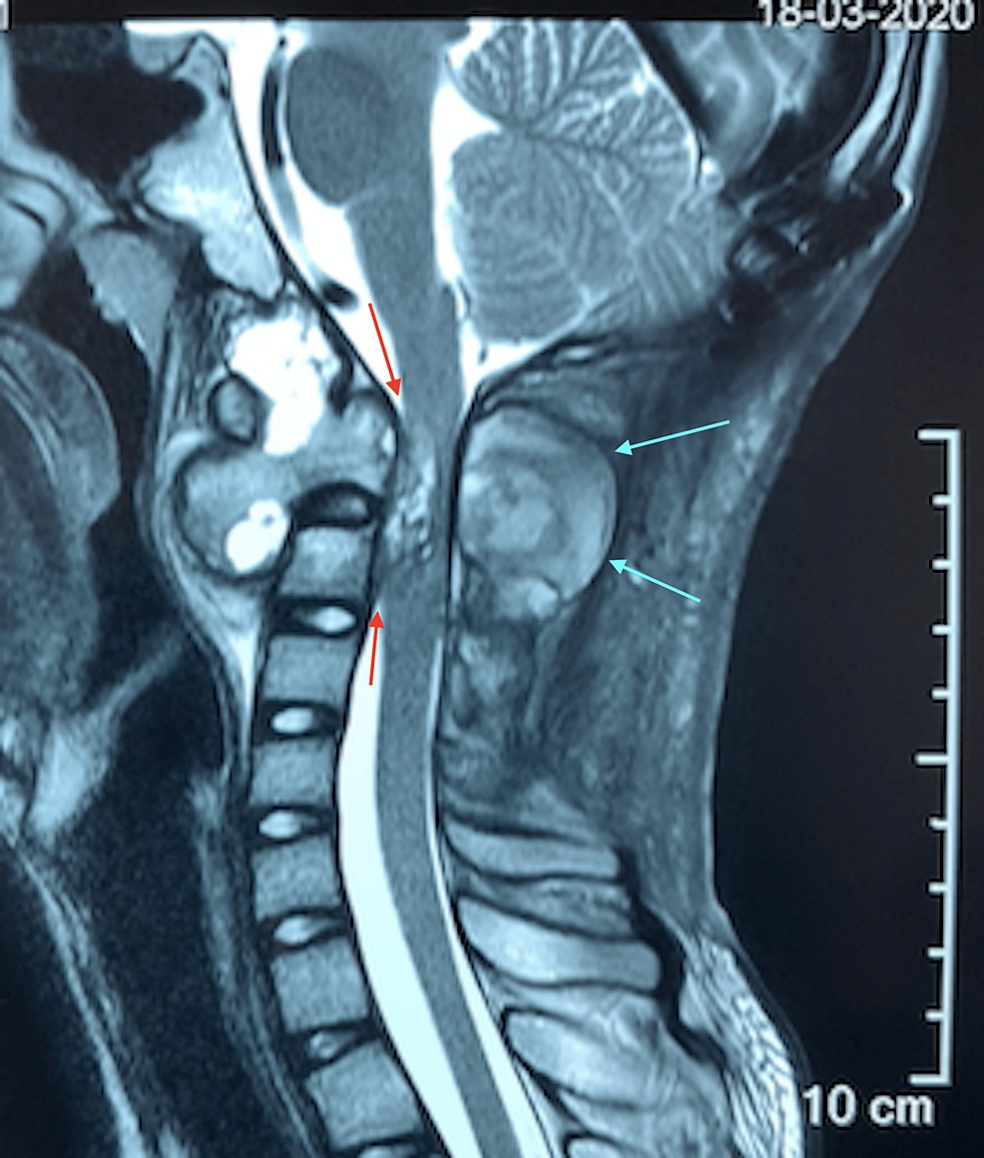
Sagittal MRI of the cervical spine after surgical excision of C2 posterior elements Compared to Figure 3, there is clearly a continued expansion of the lesion anteriorly as well as posteriorly (light blue arrows) despite the previous excision of the posterior elements of C2. This expansion of the lesion has caused more severe narrowing of the spinal canal at this level (red arrows).
